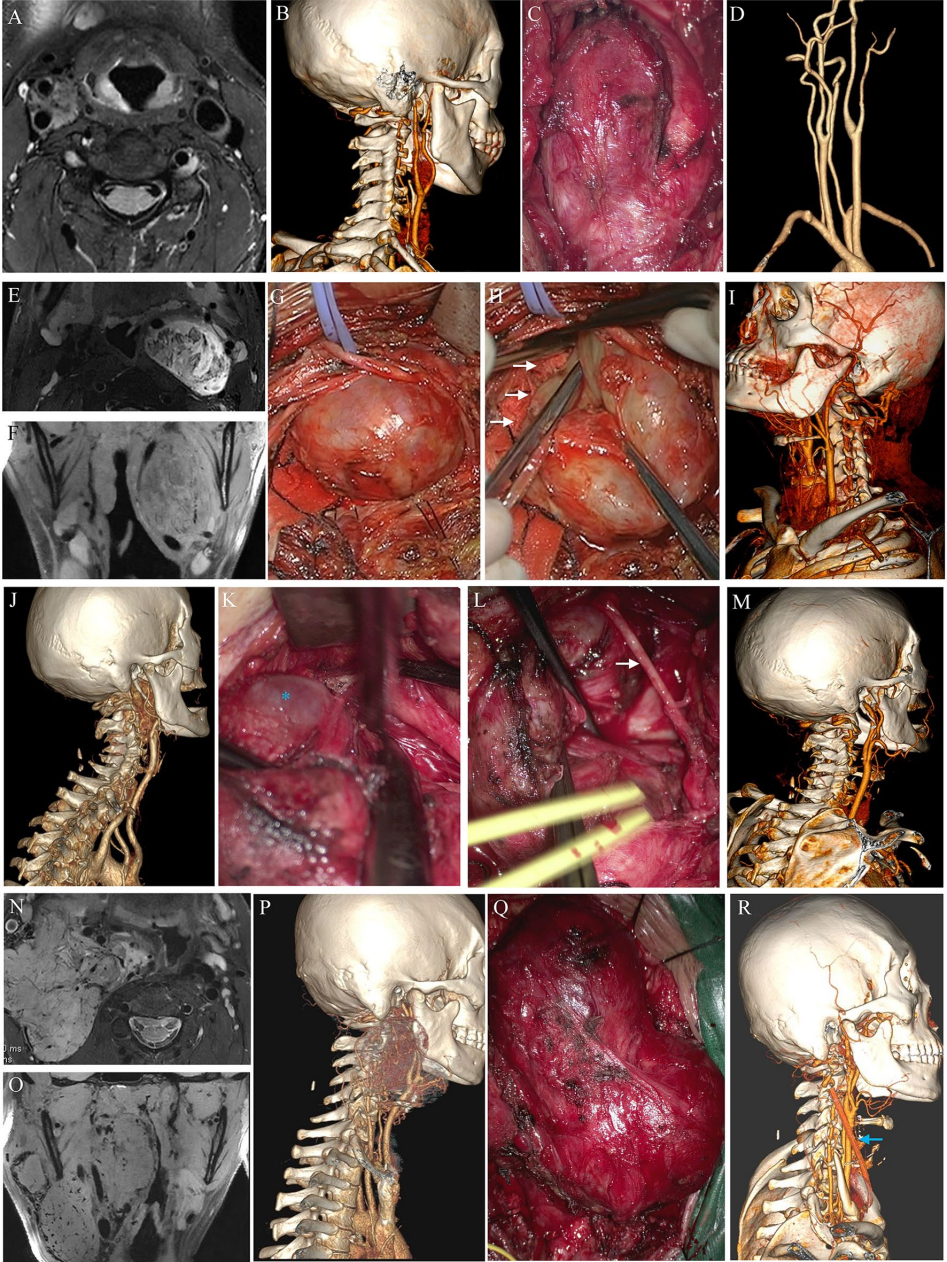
The preoperative MRI, CTA, intraoperative image and postoperative CTA images of different subtypes of tumors based on anatomical relevant classification. A-D. Common type. E-I. Pharynx-invasion type. E, F. The MRI axial and coronal view of tumor. The lateral wall of pharyngeal cavity was obviously suppressed by tumor. G, H. The main body of tumor was dragged out to expose the lateral wall of pharyngeal cavity (white arrow). Brain cotton slices were selected to protect pharynx wall. J-M. Skull base-invasion type. K. Tumor near the jugular foramen was carefully resected under high magnification. *: The jugular vein which just exits the skull base. L. The main body of tumor was dragged down to expose hypoglossal nerve (white arrow). N-R. A giant “Mixed type” of tumor invaded both the skull base and pharynx. Blue arrow: drainage tube
